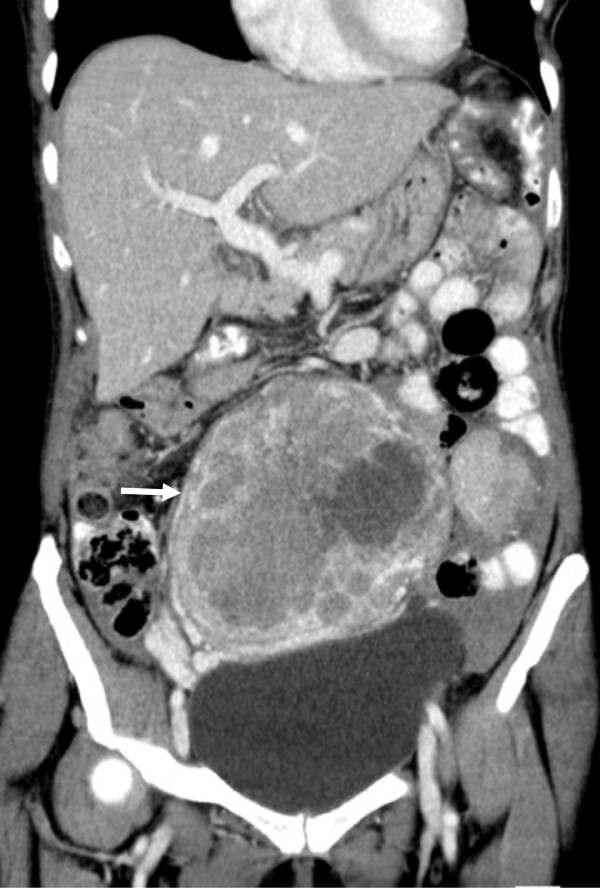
Abdominal computed tomography scan with primary tumor (14 × 10 × 12 cm) in close relation to the uterus, with peritoneal nodules and ascites (white arrow).
